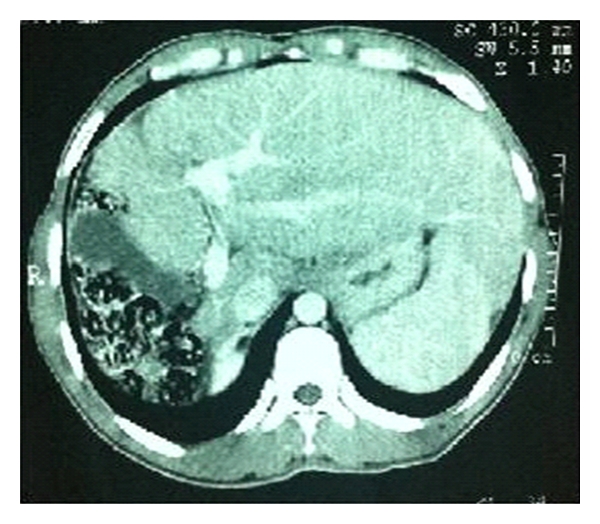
Abdominal CT: hypertrophy of the left hepatic lobe, the gallbladder is on the right of the liver in a vertical position and mild splenomegaly.
